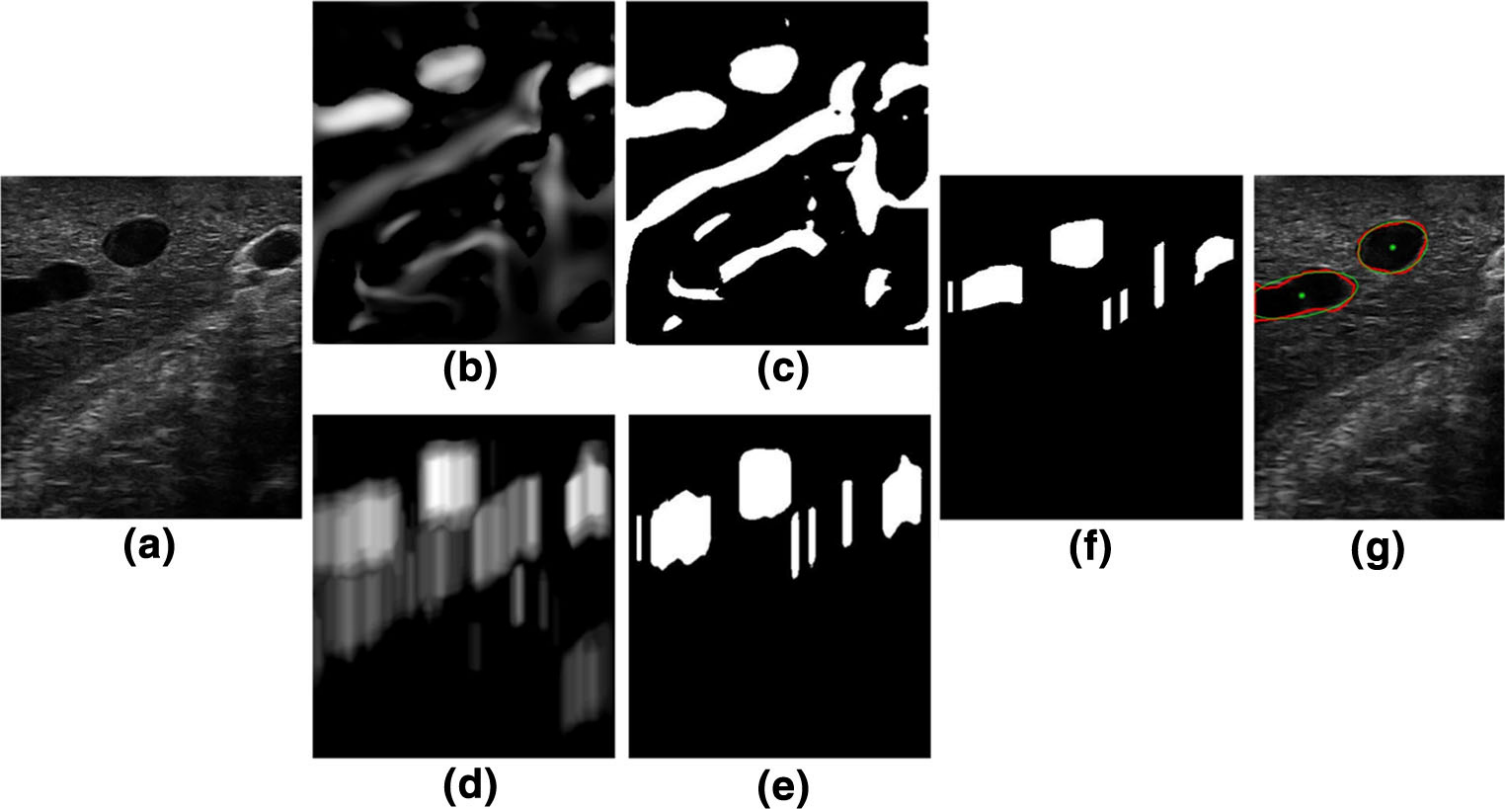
Vessel segmentation: a ultrasound B-mode image. b Vessel-enhanced image. c Thresholded vessel-enhanced image. d
